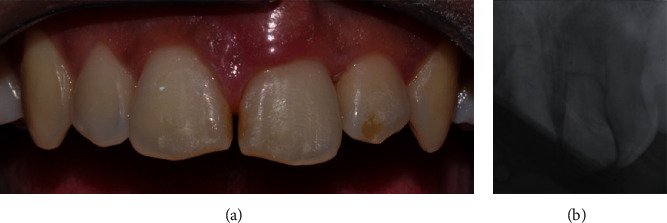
Posttraumatic (a) clinical and (b) radiographic view. Gingival tissues around the upper left central incisor are swollen and red, while a horizontal root fraction is detectable from the periapical X-ray.
